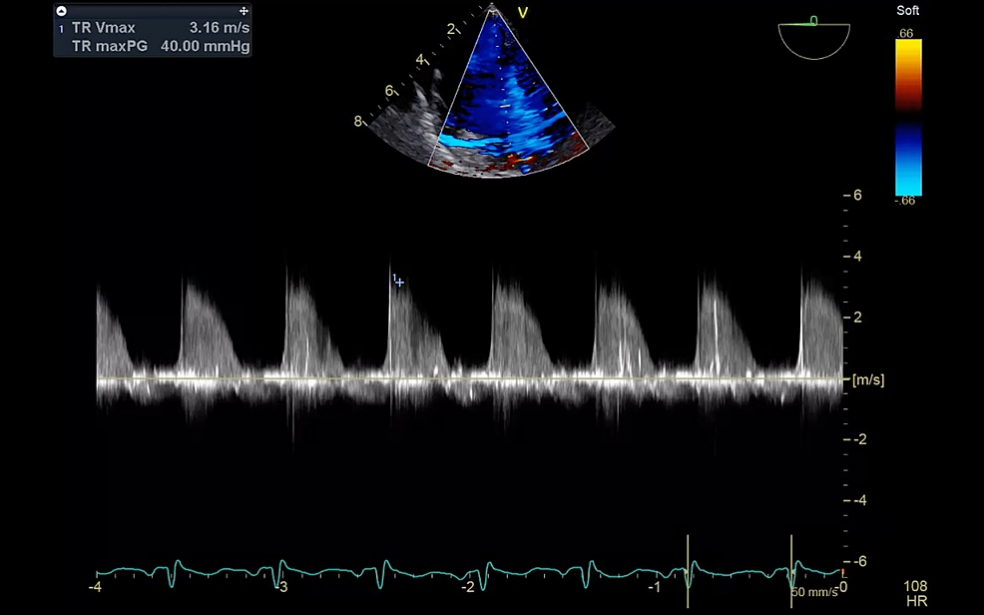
A continuous wave doppler showing early peaking signal which is suggestive of severe tricuspid regurgitation.
